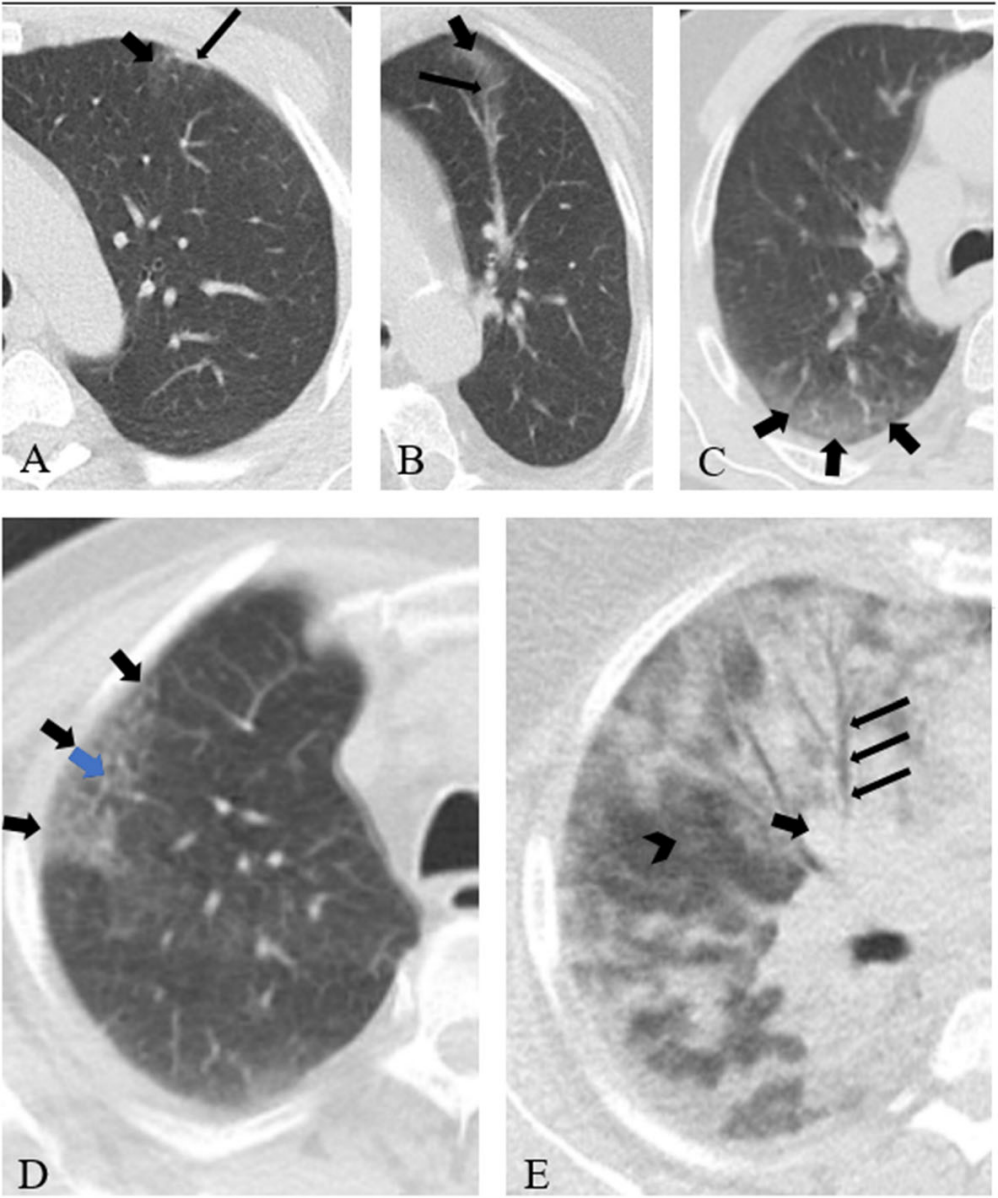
Low-dose chest CT scan images in 5 different patients with RT-PCR-confirmed COVID-19 pneumonia, showing a variety of diseased lung extension and density, comparable with the standard-dose CT scan. a Peripheral area of ground-glass opacity (thick short arrow) with overlying pleural thickening (thin long arrow). b Mixed central-peripheral lesion of ground-glass opacity (thick short arrow) with a prominent intralesional vessel (thin long arrow). c Peripheral area of ground-glass opacity (black arrows) with prominent intralesional vessels. d Peripheral area of crazy paving pattern (black arrows) with thickened interlobular septa within (blue arrow). e Mixed ground-glass opacity (arrowhead) and consolidation (thick black arrow). Long thin arrows point out air-bronchogram [images are from first author’s institution, implementing low-dose CT scan protocol, with scanning parameters as follows: kVp 120, mAs 50, pitch factor 1, thickness 3 mm]
